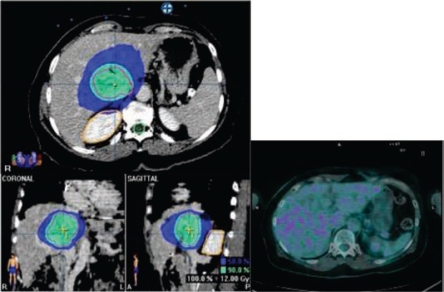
(a) stereotactic irradiation of CRC metastatis (total dose 36 Gy); (b) two-month follow-up PET/CT: no evidence of disease
